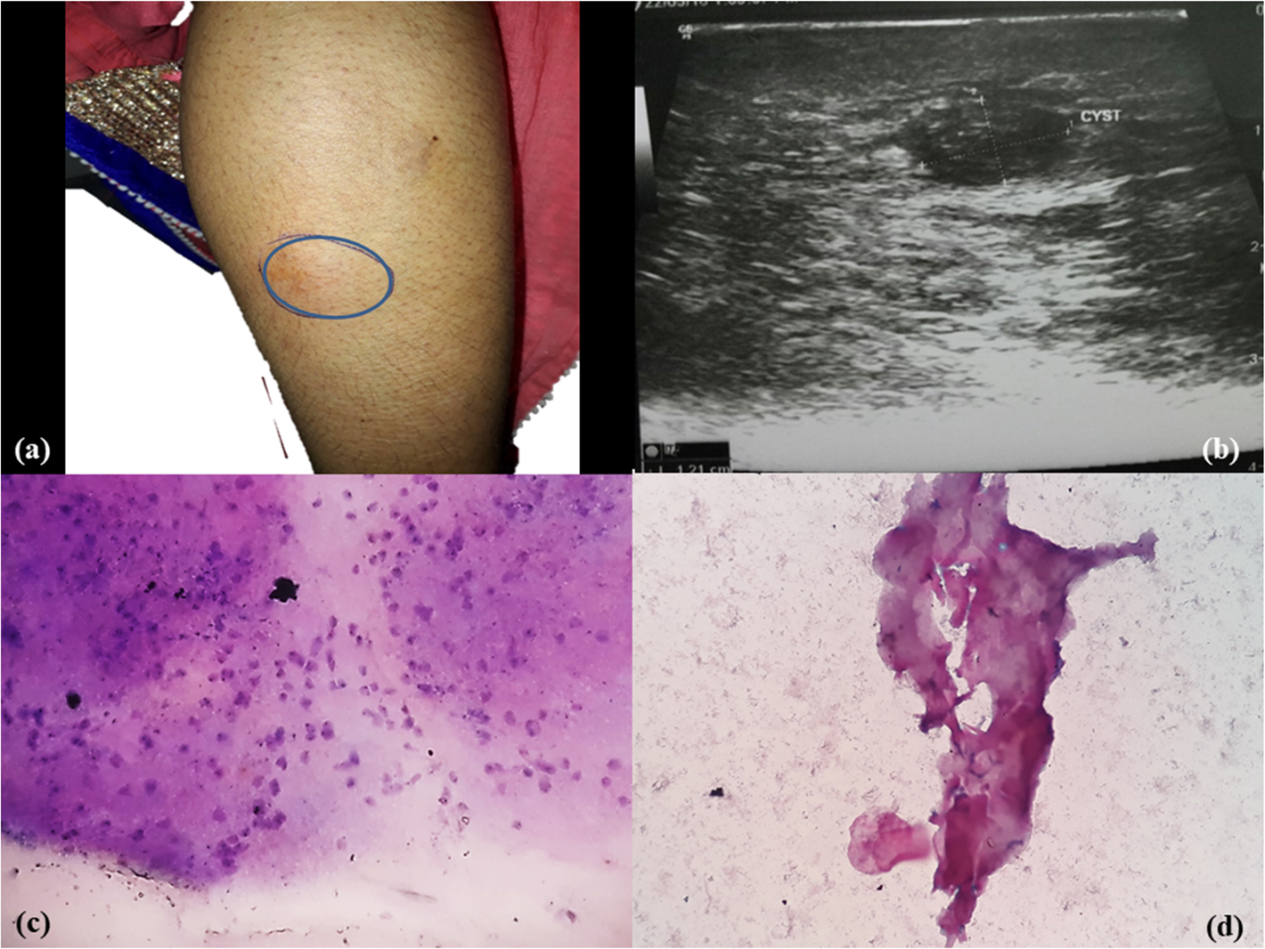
Case 3. a, b Swelling in right calf lateral aspect; ultrasonography showed a right lower limb small hypoechoic lesion in intramuscular plane measuring 1.2 × 0.7 cm. c, d Cytology showed granular bladder wall with subcuticular cells, small pyknotic-looking nuclei, fibrillary parenchyma along with inflammatory cells and homogenous acellular material. (Toluidine blue, × 40)
